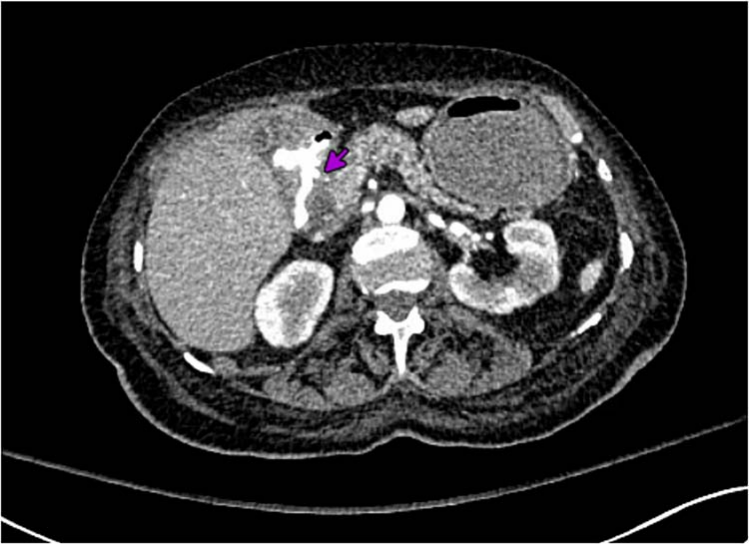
Computed tomography angiography abdomen axial view showing massive arterial bleeding in the first and second portions of the duodenum.
